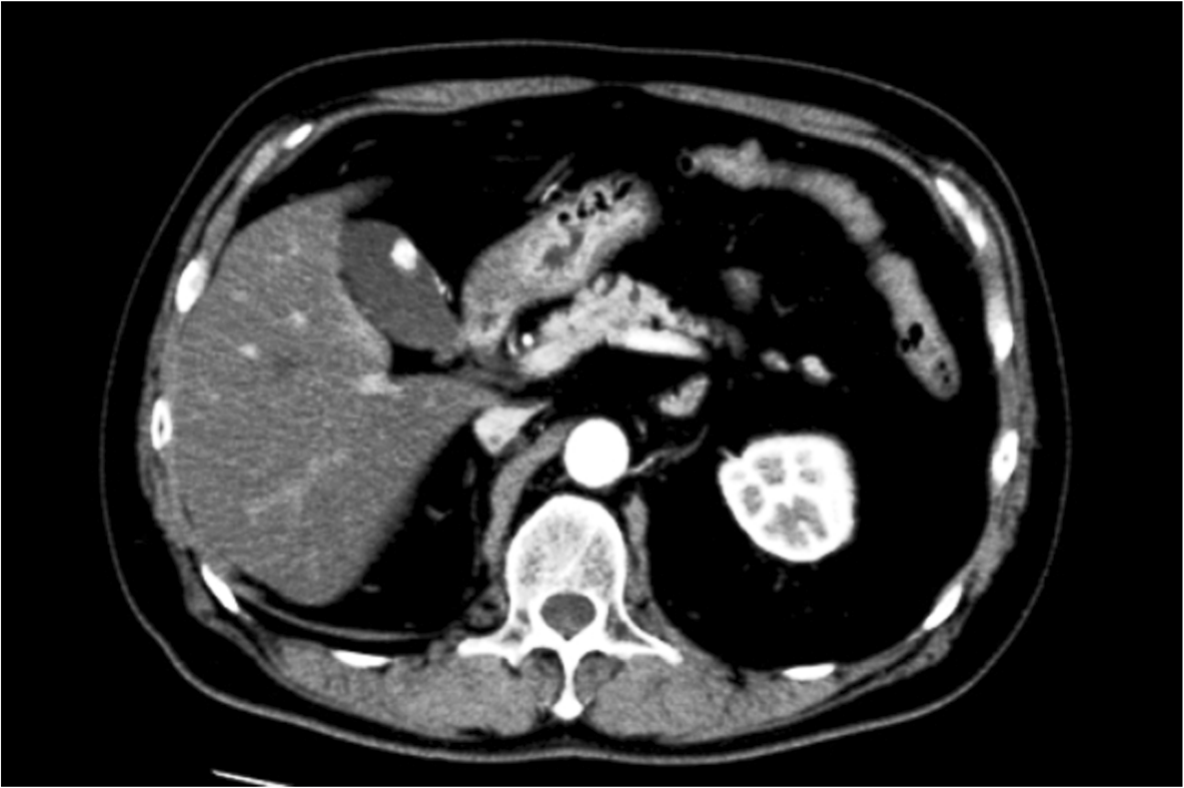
Computed tomography findings
